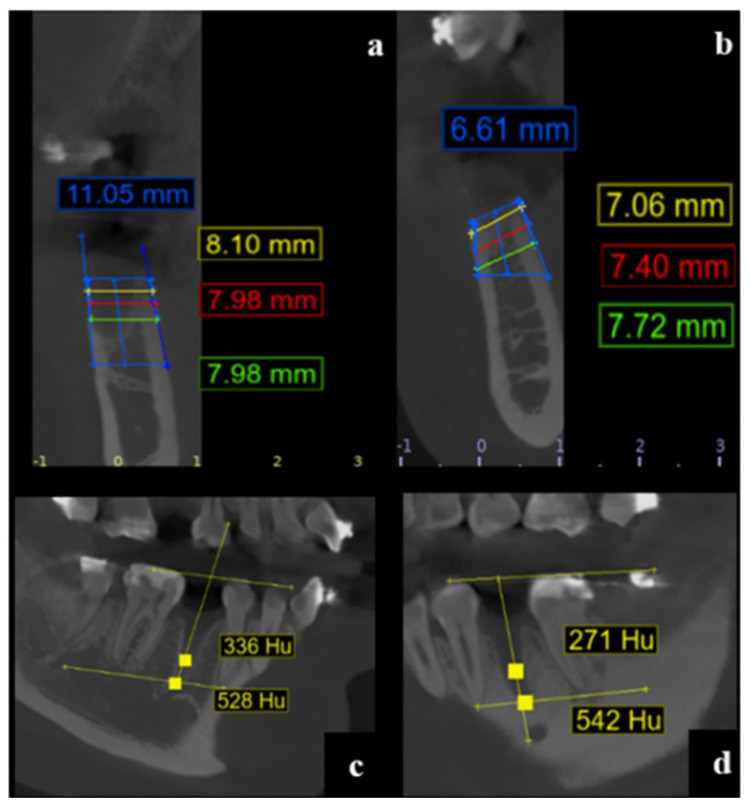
CBCT cross-sectional images at 30 days post-extraction. (a) Measurement of alveolar height and socket width at 1, 3, and 5 mm below the alveolar crest in a PRF-treated site (tooth 4.4). (b) Corresponding measurements in a control site without PRF application (tooth 3.4). (c) Hounsfield Unit (HU) assessment of bone density in the PRF-treated socket. (d) Bone density evaluation in the control socket. Measurement points are indicated with white arrows; HU values represent relative mineralization density. Color-coded lines and text indicate the measurement planes and corresponding values: blue for vertical height, red for crestal width, green for basal width, and yellow for in-termediate levels or Hounsfield Unit (HU) values.
